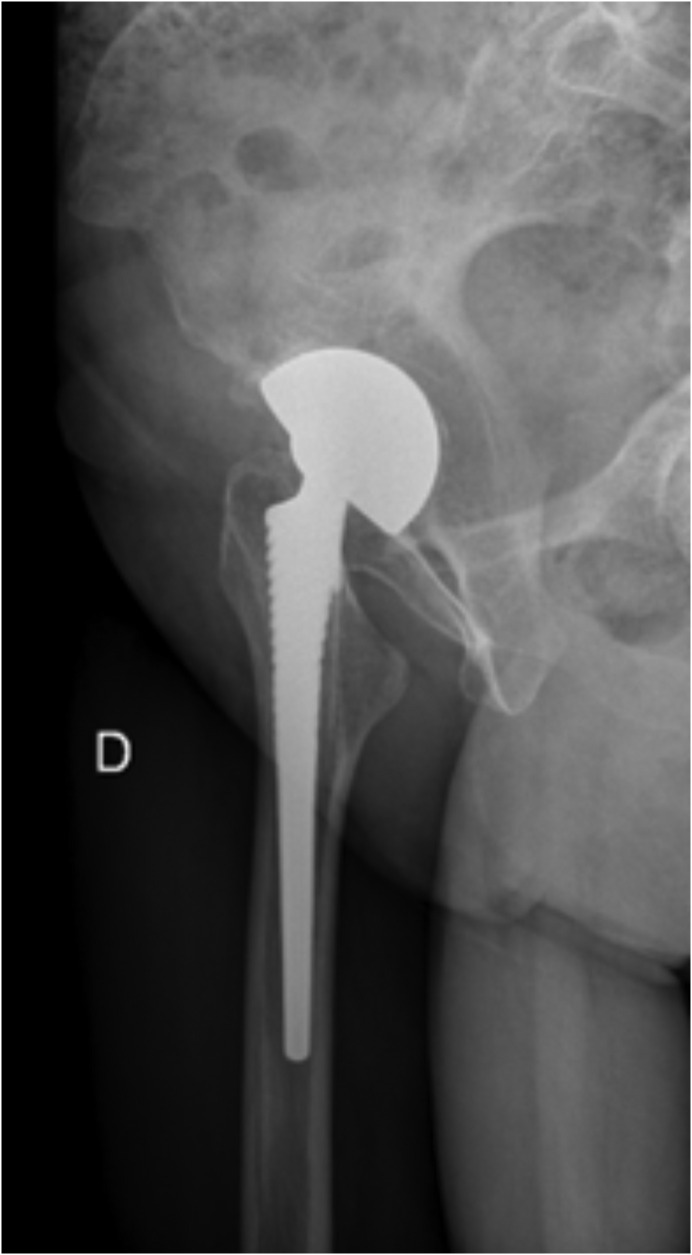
Lateral radiograph of the right total hip arthroplasty.
